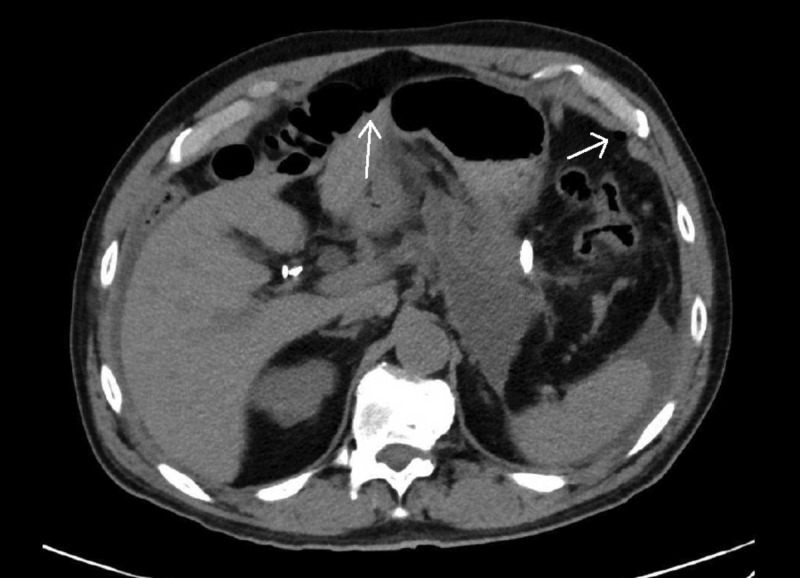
A post-EUS drainage CT abdomen (non-contrast) axial view demonstrating pneumoperitoneum (arrows) concerning for a hollow viscus perforation. EUS, endoscopic ultrasound
